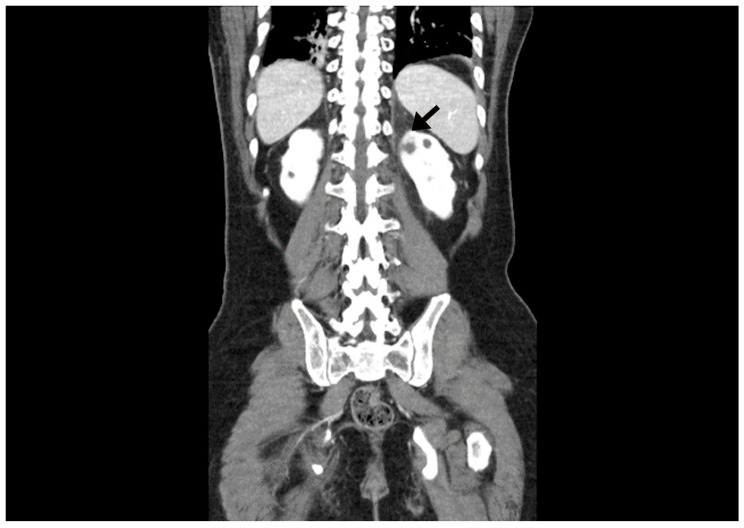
In the abdominal CT, an ill-defined low-density lesion (arrow) at the upper pole of the left kidney is noted.
